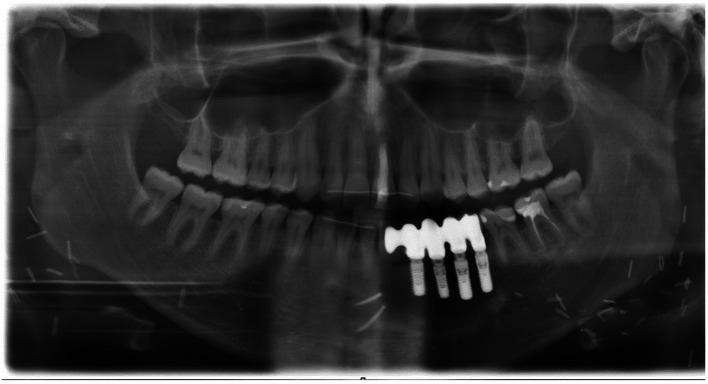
Postoperative radiological imaging: Panoramic radiography showed the final result after tumor resection and reconstruction via medial femoral condyle flap and implants in 32, 33, 34, and 35 with supraconstructions
